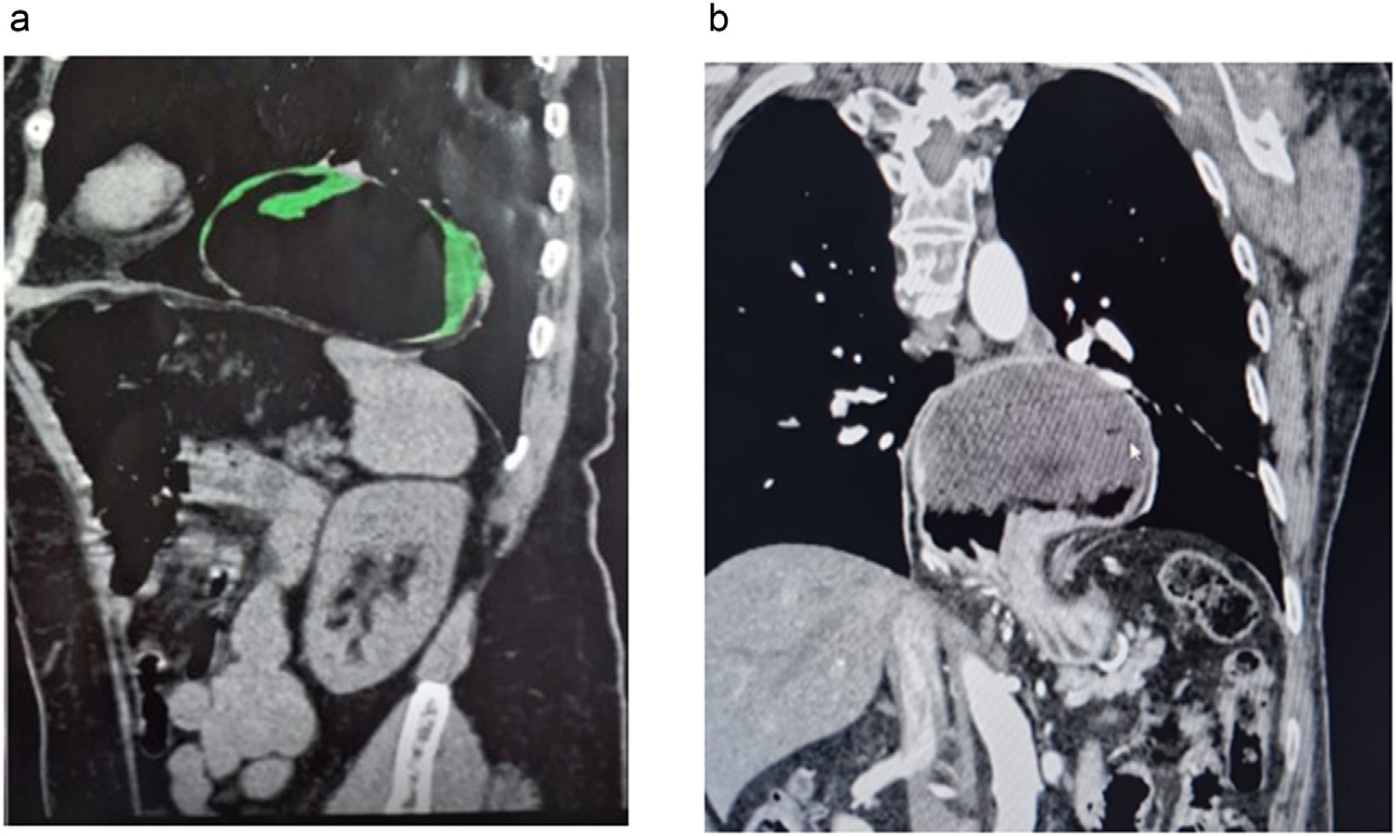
CT scan (right) showing sagittal and coronal aspect of large hiatal hernia with significant intrathoracic stomach and esophageal displacement.
